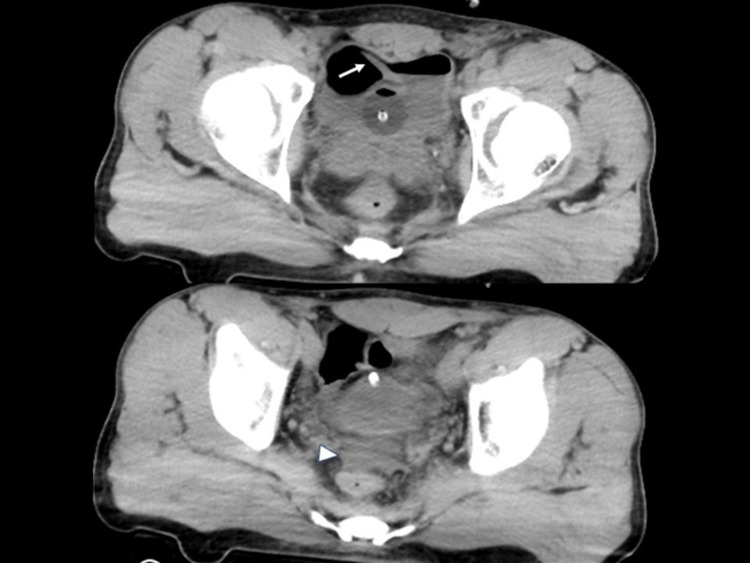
Computed tomography (CT) findings on arrival CT revealed trabecular formation in the bladder (arrow) and slight fluid collection in the rectovesical pouch (triangle).
